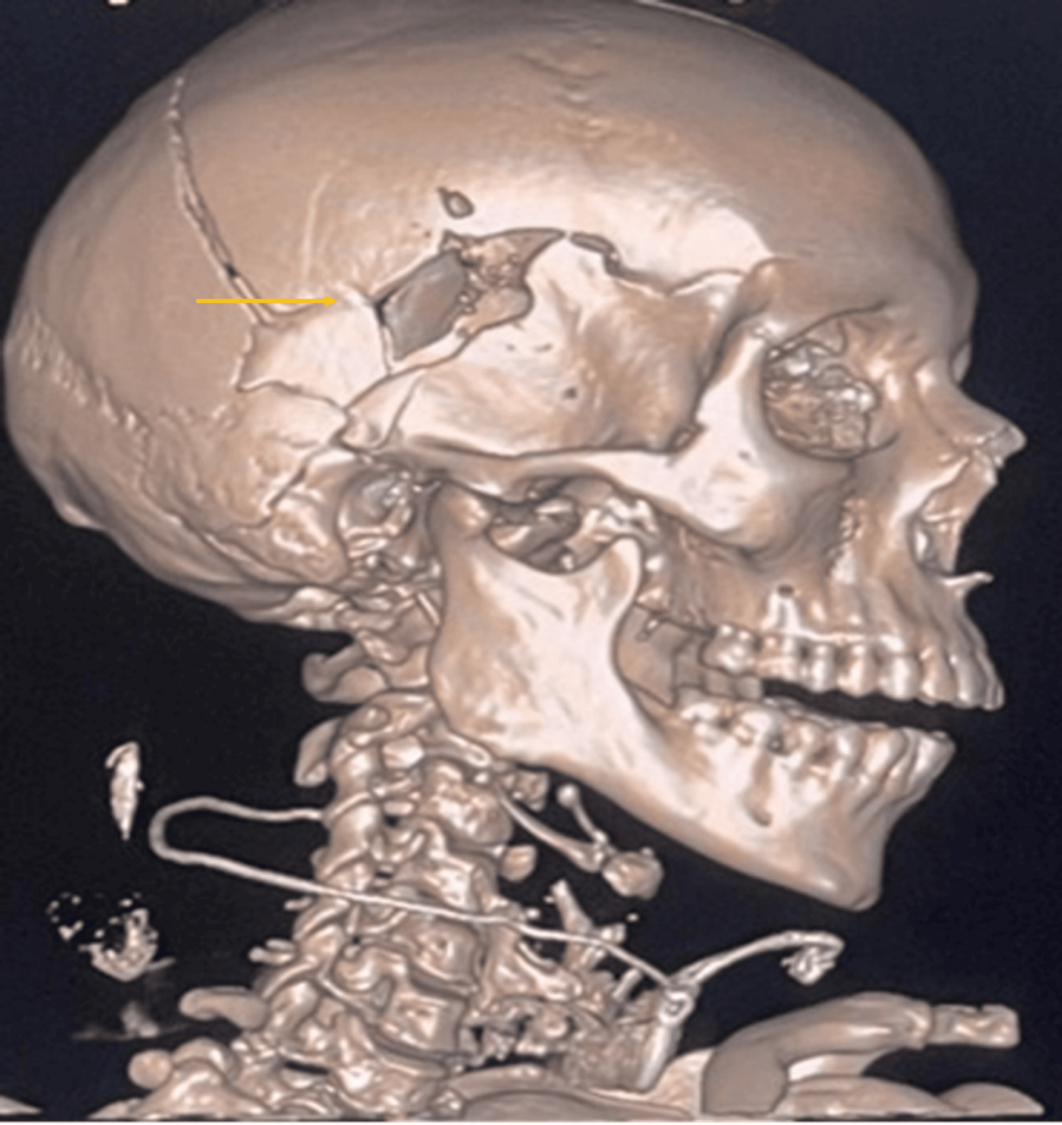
CT image of the cranial trauma showing a gunshot entry wound in the right temporal region
